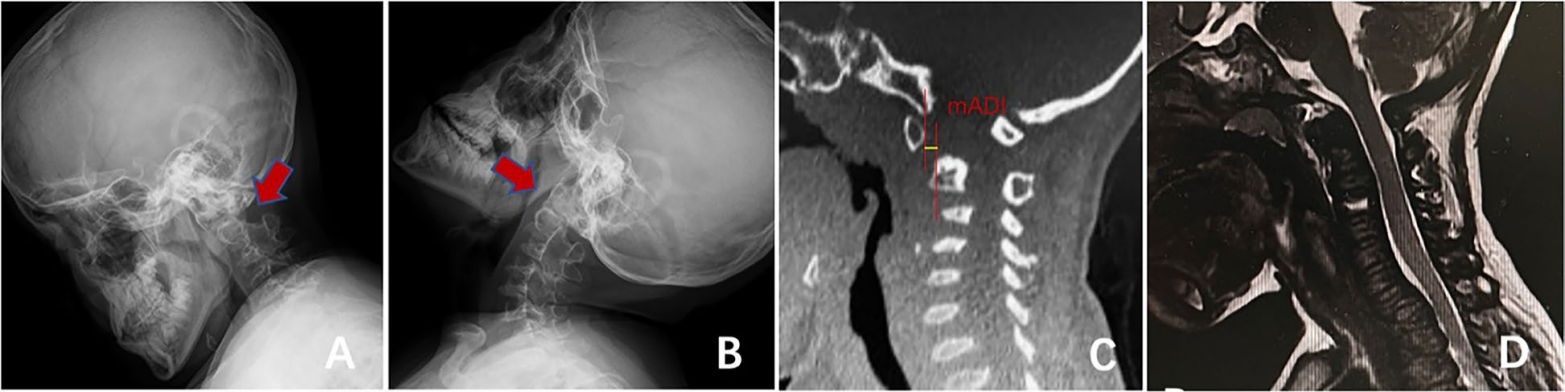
Imaging findings of an 8-year-old girl who was diagnosed with type IVA MPS. Dynamic radiograph suggesting atlantoaxial instability (A, B). CT showing the absence of odontoid process and flattened vertebrae with less smooth edges (mADI value = 5.5 mm) (C). MRI showing no obvious stenosis of the spinal canal, no compression of the cervical spinal cord, and no abnormal signal. Since 2021, this patient has been regularly injected with sulfatase every week and is now in the process of regular follow-up (D)
